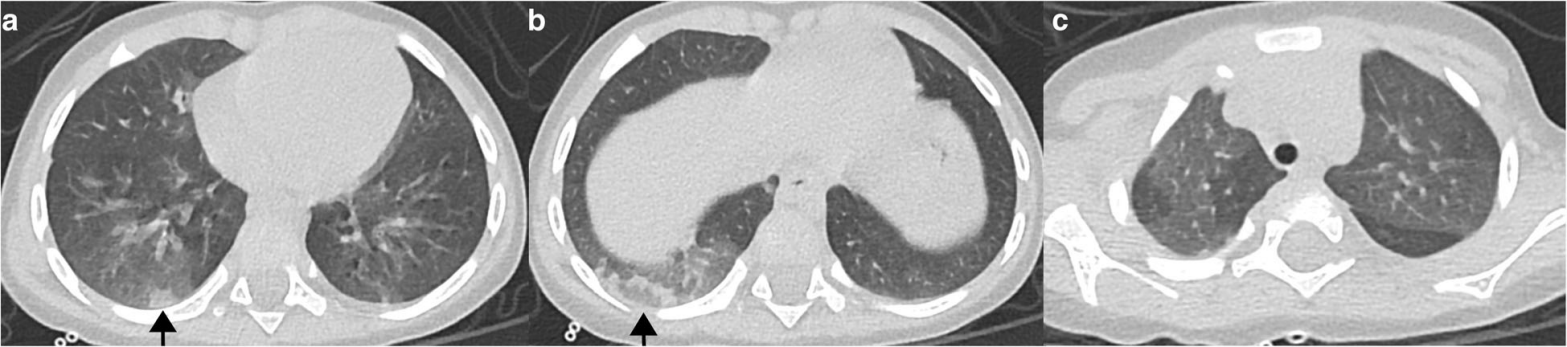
Typical findings in a 1-year-old ex-premature girl. CT shows small subpleural consolidation with surrounding ground-glass opacity (halo sign—arrow in a), perilobular peripheral opacity (arrow in b), suggestive of secondary organizing pneumonia, with underlying ground-glass opacities. Mosaic attenuation pattern was also seen (c) suggesting concomitant small airways disease (probably due to coinfection with other viral diseases, in this case, metapneumovirus)
